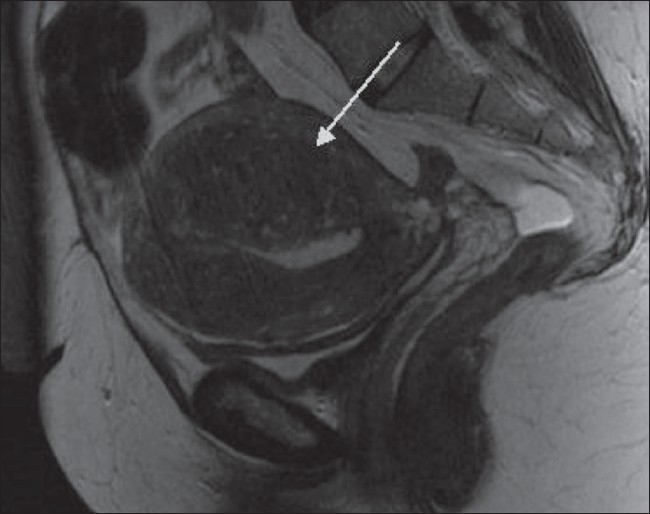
Sagittal T2W MRI image shows thickening of the junctional zone in a patient with adenomyosis. The thickening is most marked posteriorly (arrow) and several small cystic spaces can be seen within it
